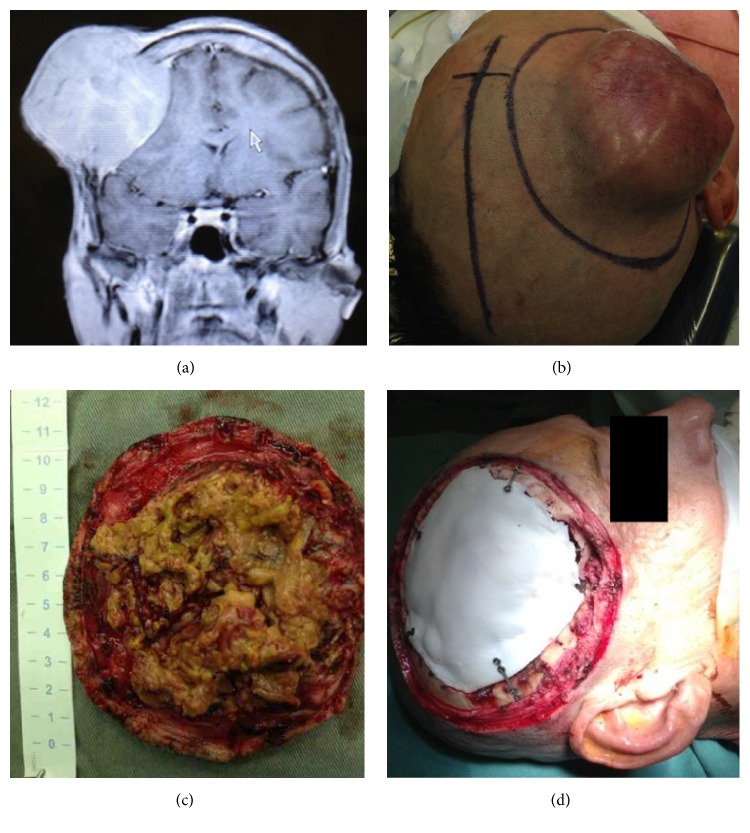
Intraoperative photos showing. (a) Brain MRI sequence with GD in T1 showing lesion with homogeneous enhancement contrast in scalp associated with bone invasion and compressive effect on the adjacent brain parenchyma. The mass enhanced homogeneously. (b) Delimitation of skin incision around the tumor on the right, midline delimitation on the left. (c) Tumor measures after the tumor resection. (d) Cranioplasty with bone cement.
